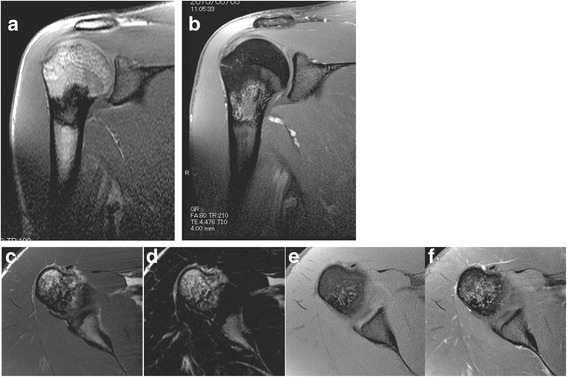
a–f MRI scans of the right shoulder at initial presentation. T1-weighted coronal (a), T1-weighted transverse (c), and T2-weighted fat-suppressed (e) images of the proximal humeral lesion showing primarily low to intermediate signal intensities with some small foci of high signal intensity. T2-weighted coronal (b), T2-weighted transverse (d), and with gadolinium contrast (f) images of the proximal humeral lesion showing primarily high signal intensities with some streaks of low signal intensity
